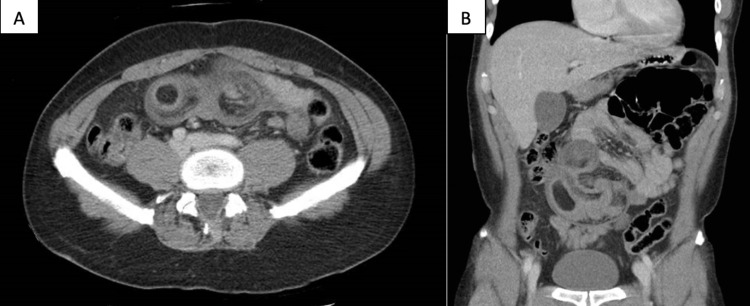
CT imaging. Axial view (A) and coronal view (B) of intussusception within the mid-abdomen with edematous and dilated bowel, compatible with obstruction. The lead point is suspected to be a 3.9 cm small bowel lipoma. No free intraperitoneal air or fluid collection.
